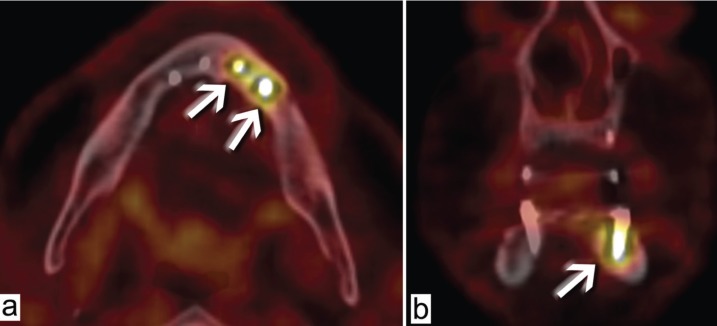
Patient 3. FDG PET/CT fusion images in horizontal (a) and frontal (b) sections. Positions 31 and 33 (arrows) show peri-implant hypermetabolism, whereas positions 41 and 43 are metabolically normal.
